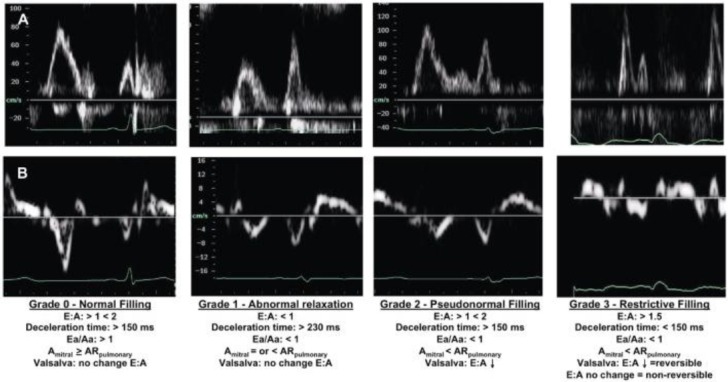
Grades of diastolic dysfunction by means of pulsed wave mitral Doppler and tissue Doppler mitral annular measurement [17]*.
